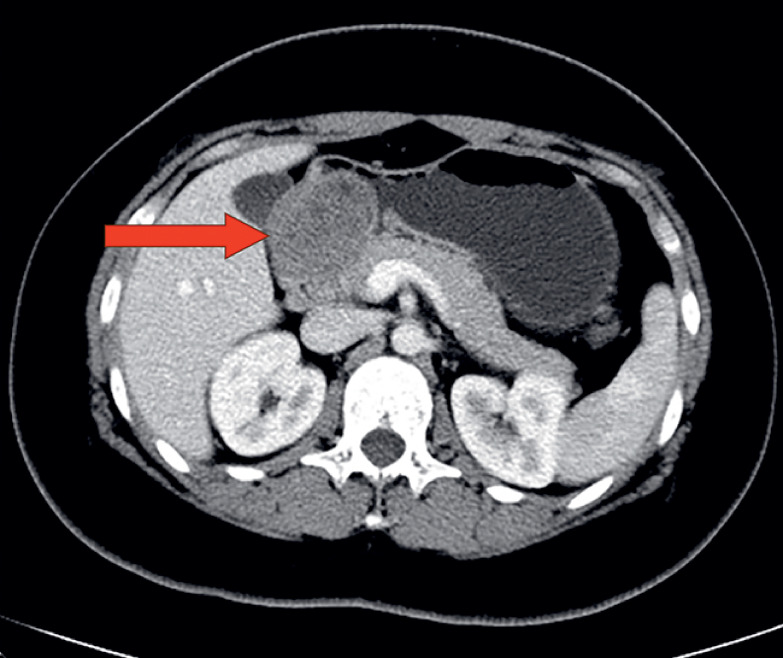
The submucosal tumor (red arrow) at the posterior wall of antrum on computed tomography.
